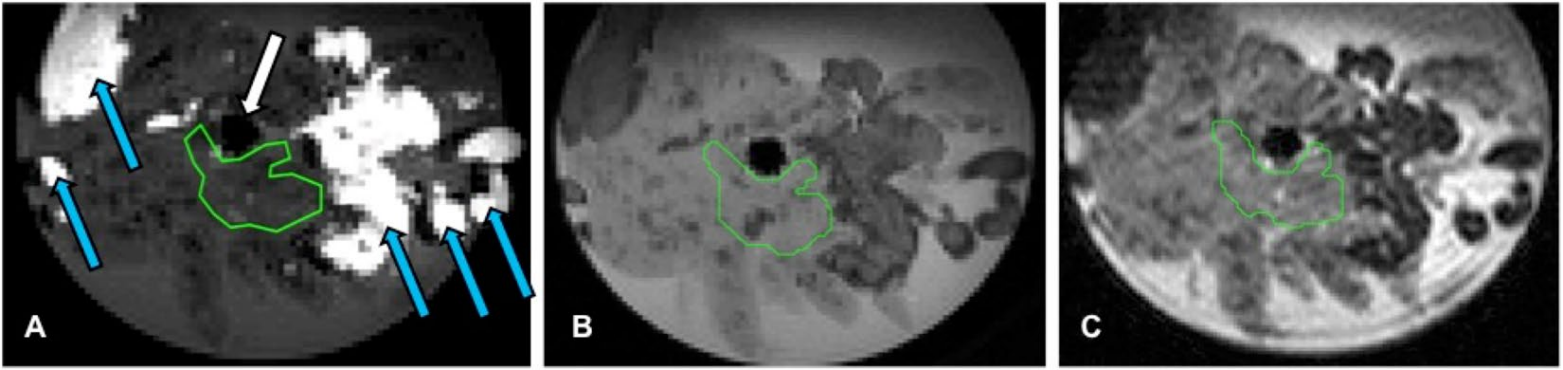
Representative images of a pancreas explant at MRI. These images are acquired in a horizontal plane across the circular sample holder, and form the basis for the computation of the quantitative MRI parameters described in the methods section. (A) Magnitude image obtained with the MR elastography sequence (spatial resolution: 300 µm, no filtering applied, repetition time: 1000 ms, echo time: 18 ms, flip angles: 90°−180°). The mechanical transducer is visible as a dark region at the center of the sample (white plain arrow). Pancreatic parenchyma appears as dark grey, whereas fatty tissue is visible as bright areas (blue arrows). The region of interest used for reporting quantitative results (yellow outline) is positioned close to the mechanical transducer and inside the pancreatic parenchyma. (B) Magnitude image obtained with the chemical shift-encoded gradient-echo MR imaging sequence (spatial resolution: 250 µm, no filtering applied repetition time: 950 ms, only a single echo time of 1.65 ms is displayed, flip angle: 15°). The region of interest (yellow outline) is co-registered to the region of interest selected for MR elastography. On this contrast, the pancreatic parenchyma appears light grey, whereas the fatty tissue appears as dark grey. (C) Magnitude image obtained with the diffusion weighted imaging sequence (spatial resolution: 195 µm, no filtering applied, repetition time: 2000ms, echo time: 20 ms, flip angles: 90°−180°, fat suppression applied). Only the first b-value (b = 0 s/mm²) is displayed. The region of interest (yellow outline) is co-registered to the region of interest selected for MR elastography. On this contrast, the pancreatic parenchyma appears as light grey, whereas the fatty tissue appears as very dark gray due to the fat suppression.
